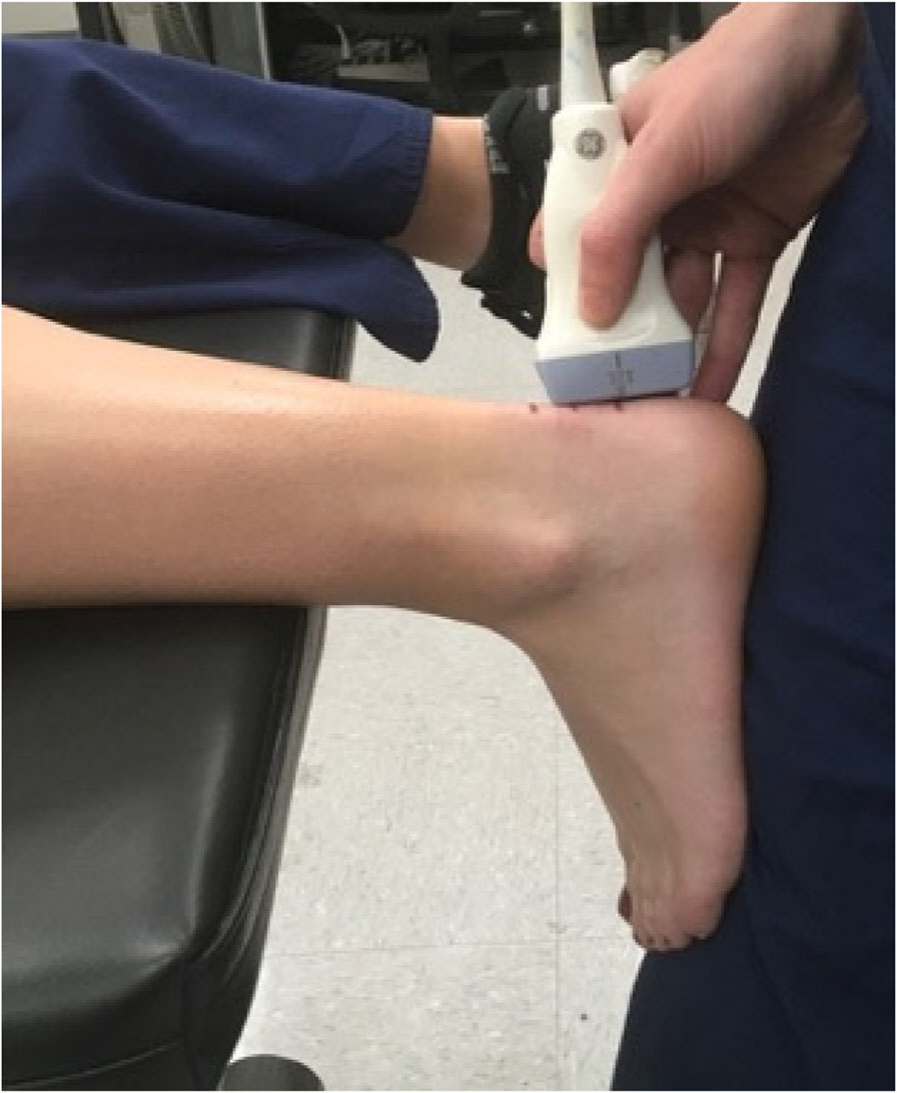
Placement of the ultrasound transducer
